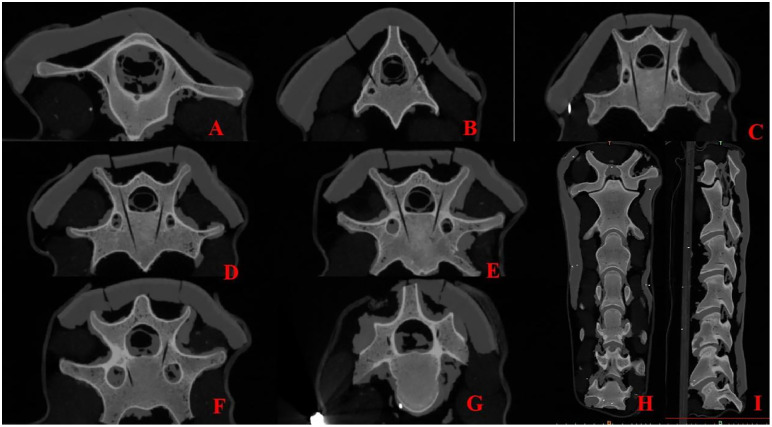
Postoperative CT Images of the Needle-Removed Skeleton. A-G Postoperative vertebrae (C1-C7) in the horizontal plane； H Vertebrae in the coronal plane； I Vertebrae in the sagittal plane.
